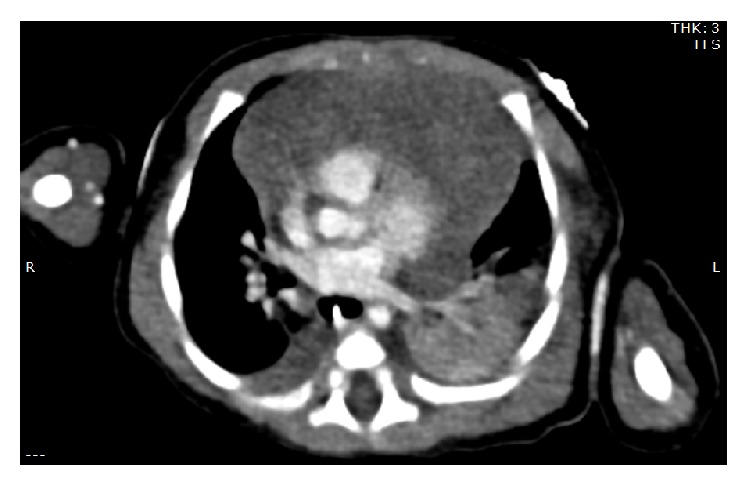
CT of chest/abdomen/pelvis demonstrated a homogeneously enhancing anterior mediastinal mass which maintained thymic contour, with mass effect upon the vessels and airway.
